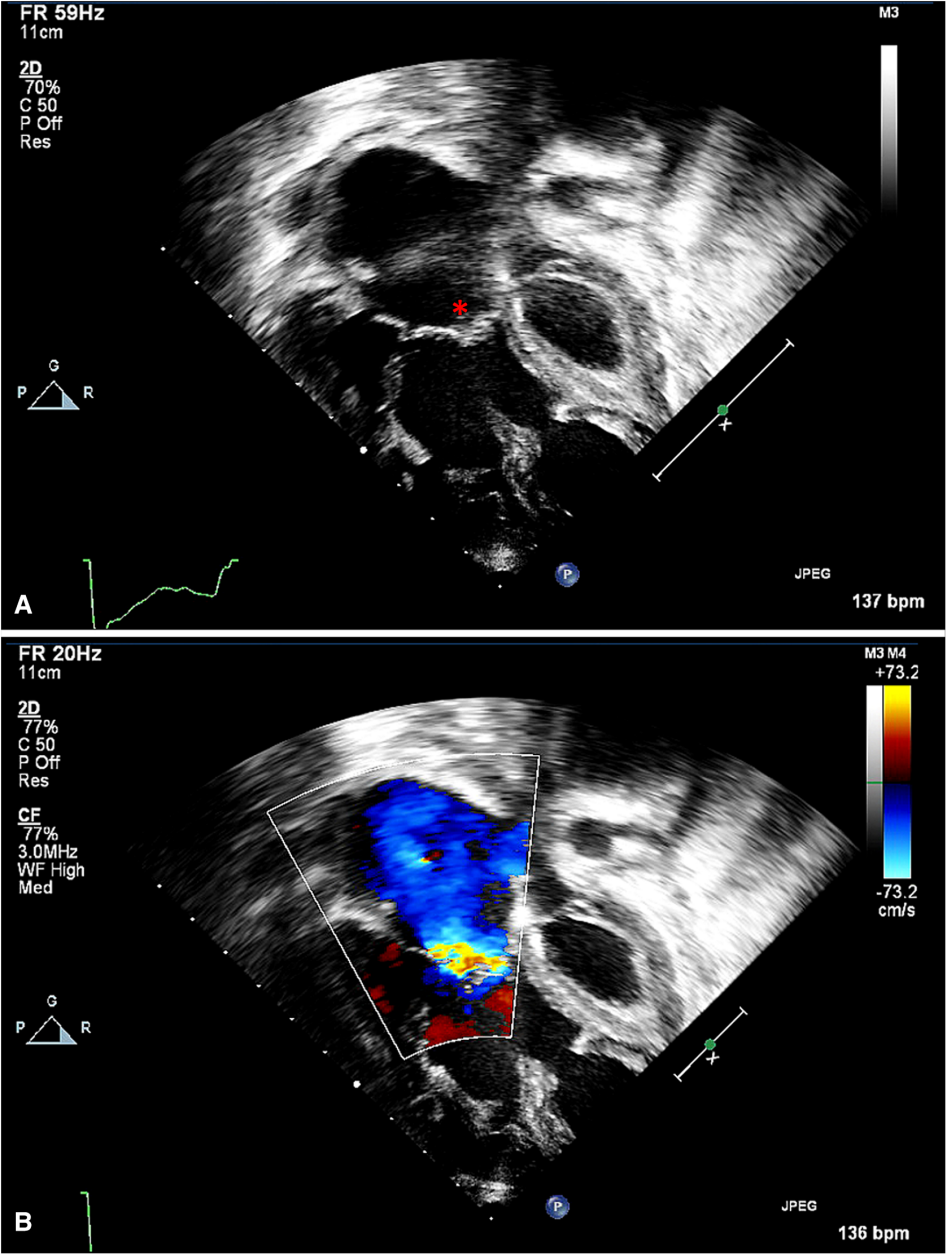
Apical view of tricuspid valve, with annular dilatation. (A) The valve's coaptation area is reduced (*). (B) The color Doppler interrogation indicates a broader area of regurgitation, through multiple fenestrations in addition to the central area of malcoaptation.
